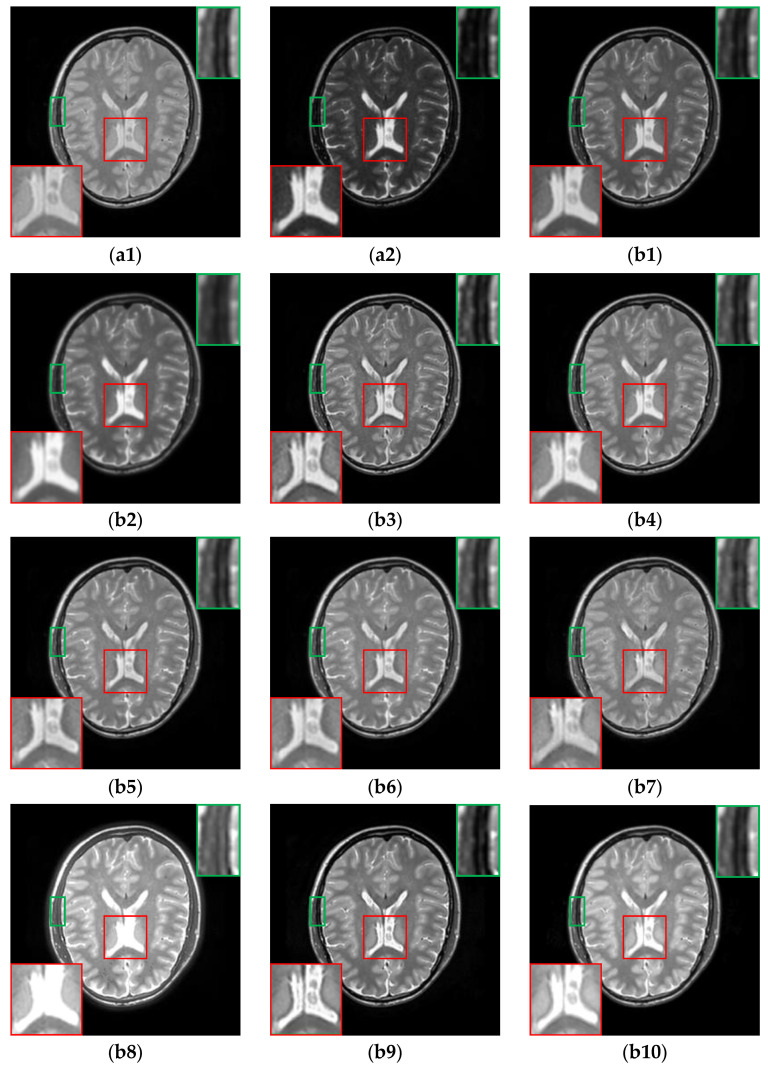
The results of all algorithms on the multi-modal MR image pairs from IXI dataset. (a1) Source PD image; (a2) Source T2 image; (b1) U2Fusion; (b2) DenseFuse; (b3) IFCNN; (b4) NestFuse; (b5) RFN-Nest; (b6) PAPCNN; (b7) ReLP; (b8) TIF; (b9) CDDFuse; (b10) CIRF.
